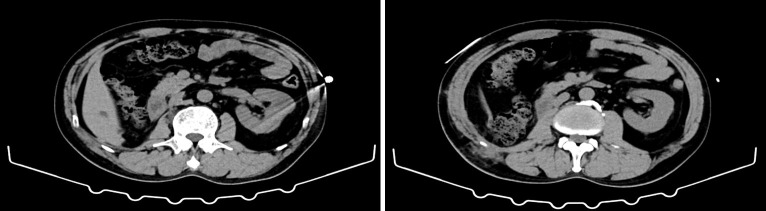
Non-contrast follow-up CT (axial view) 2 months after surgery: The right kidney is absent postoperatively, and postoperative scar changes are noted in the right lumbar region. The anatomy at the operative site is mildly distorted, with strand-like linear densities. Several small lymph nodes are seen in the abdominal cavity and retroperitoneum. No definite intra-abdominal or retroperitoneal fluid collection is identified.
